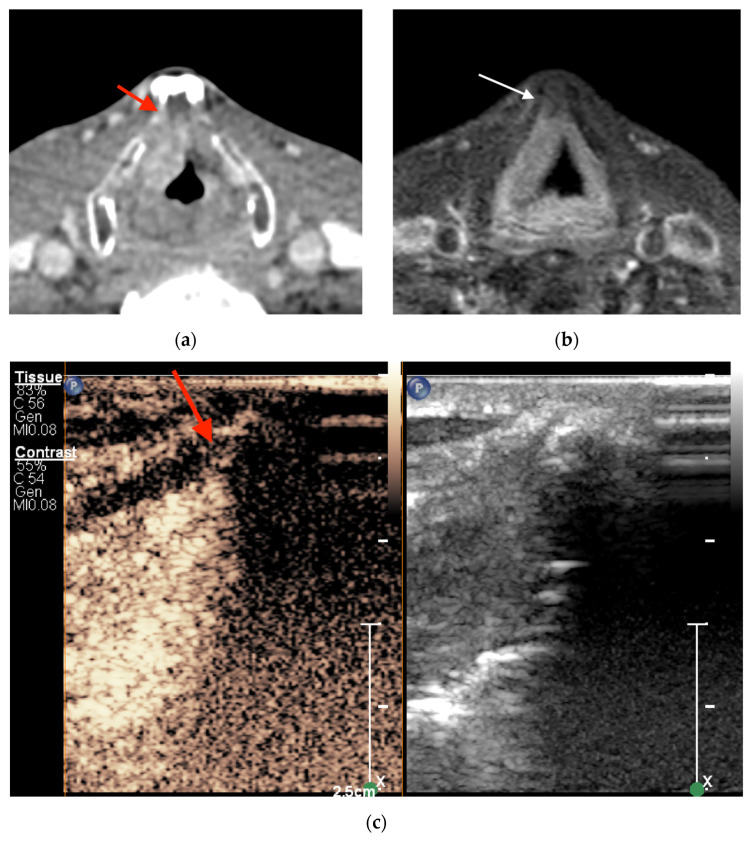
Supraglottic squamous cell carcinoma on the right side anteriorly adjacent to non-ossified cartilage inner lamina. (a) Axial CECT and (c) CEUS findings were false positive (red arrow) for tumor invasion of the thyroid cartilage, whereas MRI findings, as shown in (b), in axial contrast-enhanced high-resolution T1-weighted turbo spin echo Dixon images were true negative (white arrow).
